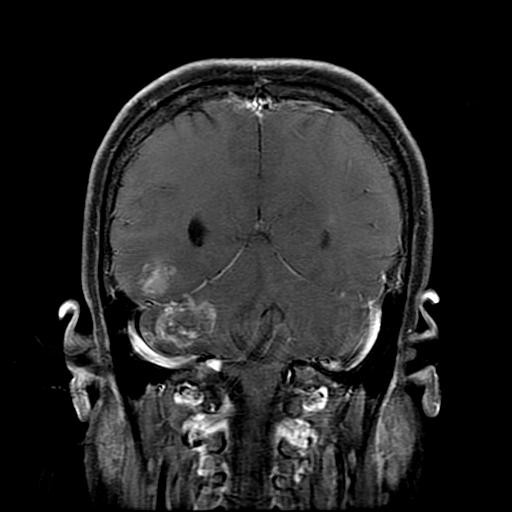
Brain MR imaging showed multiple heterogeneously enhanced lesions. The largest lesion, seen in the right cerebellar hemisphere, was later surgically resected.
